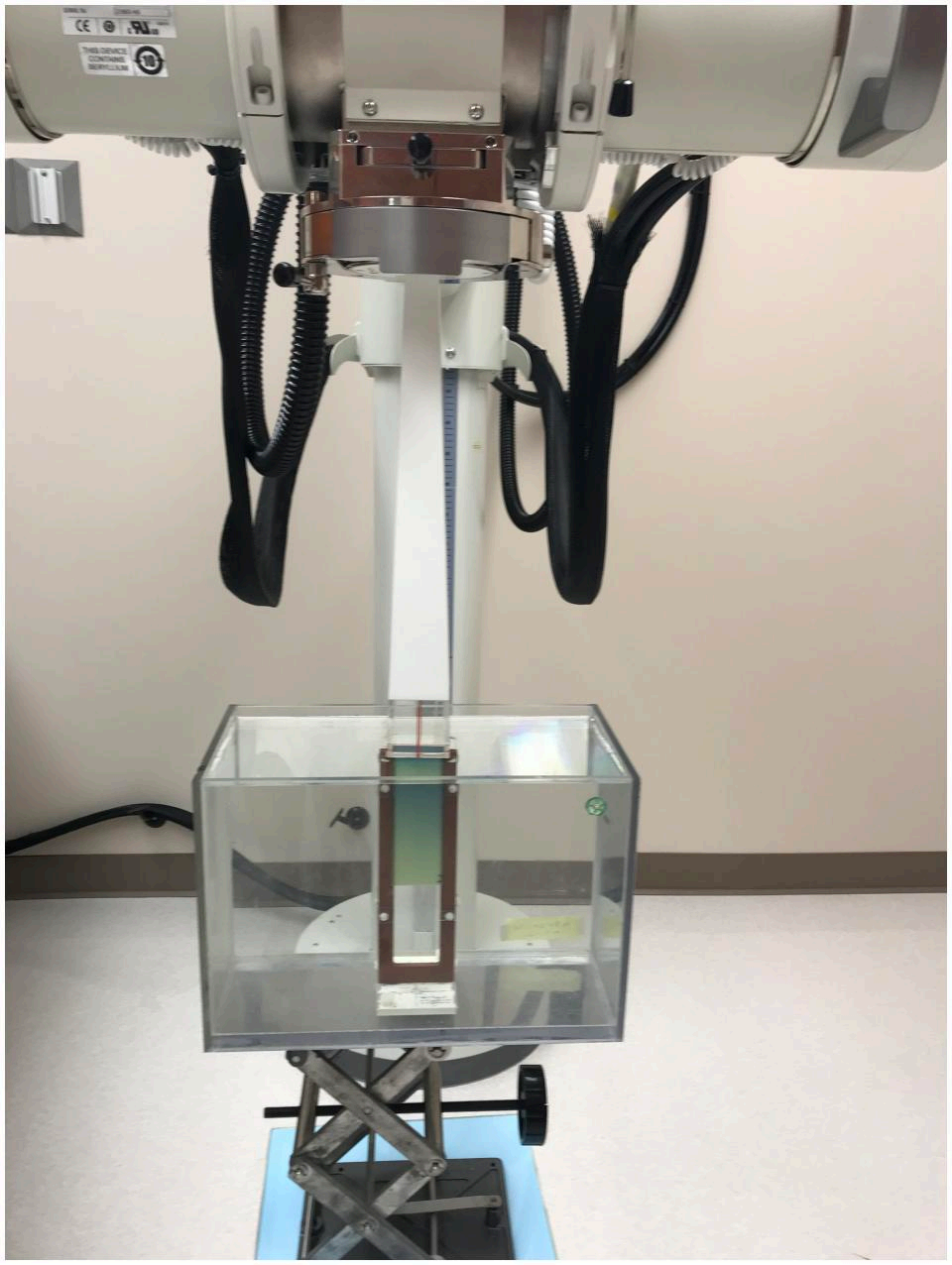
Setup for percentage depth dose measurements with film in water on the Xstrahl 300 kilovoltage x-ray therapy unit.
